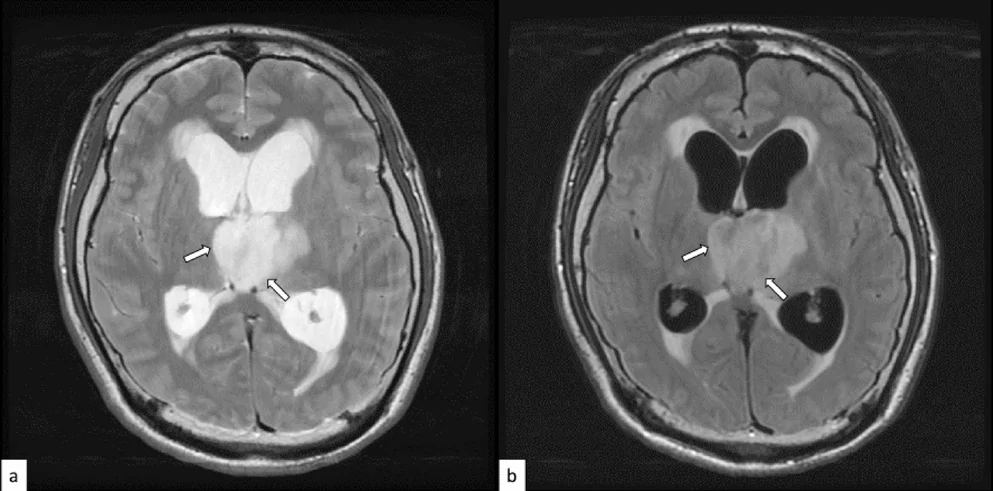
Diffuse midline glioma, H3 K27-altered in a 36-year-old man. The thalamic tumor mass shows hyperintensity on T2-weighted image (a) and hypointensity on fluid-attenuated inversion recovery (FLAIR) image (b). The T2-FLAIR mismatch is observed in this case
